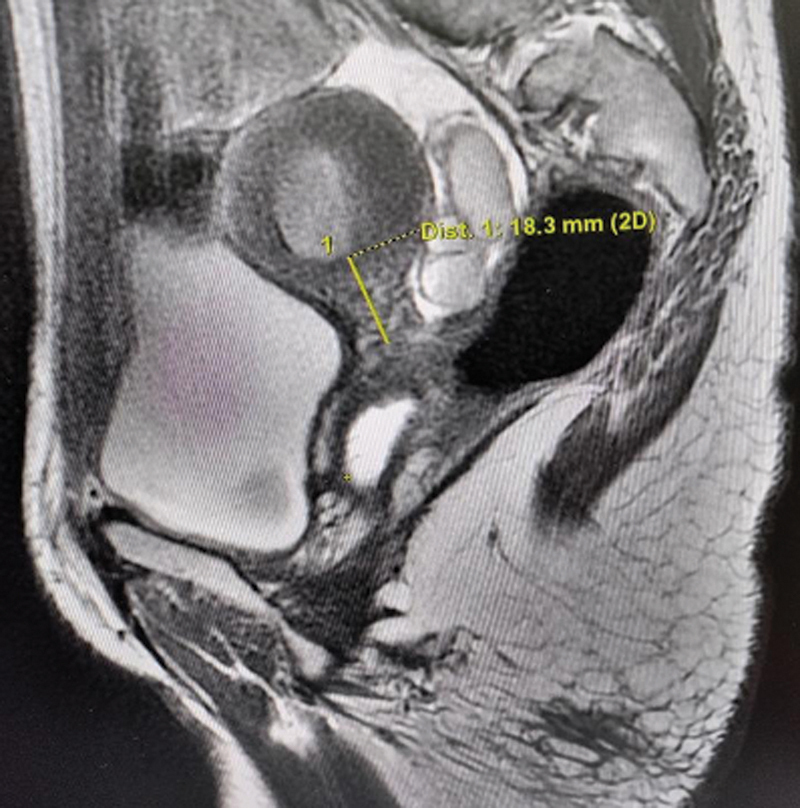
T2-weighted magnetic resonance imaging showing hematometra with an 18.3-mm long noncanalized cervix. Saline was injected in the vagina prior to imaging to delineate the cervicovaginal relationship.
